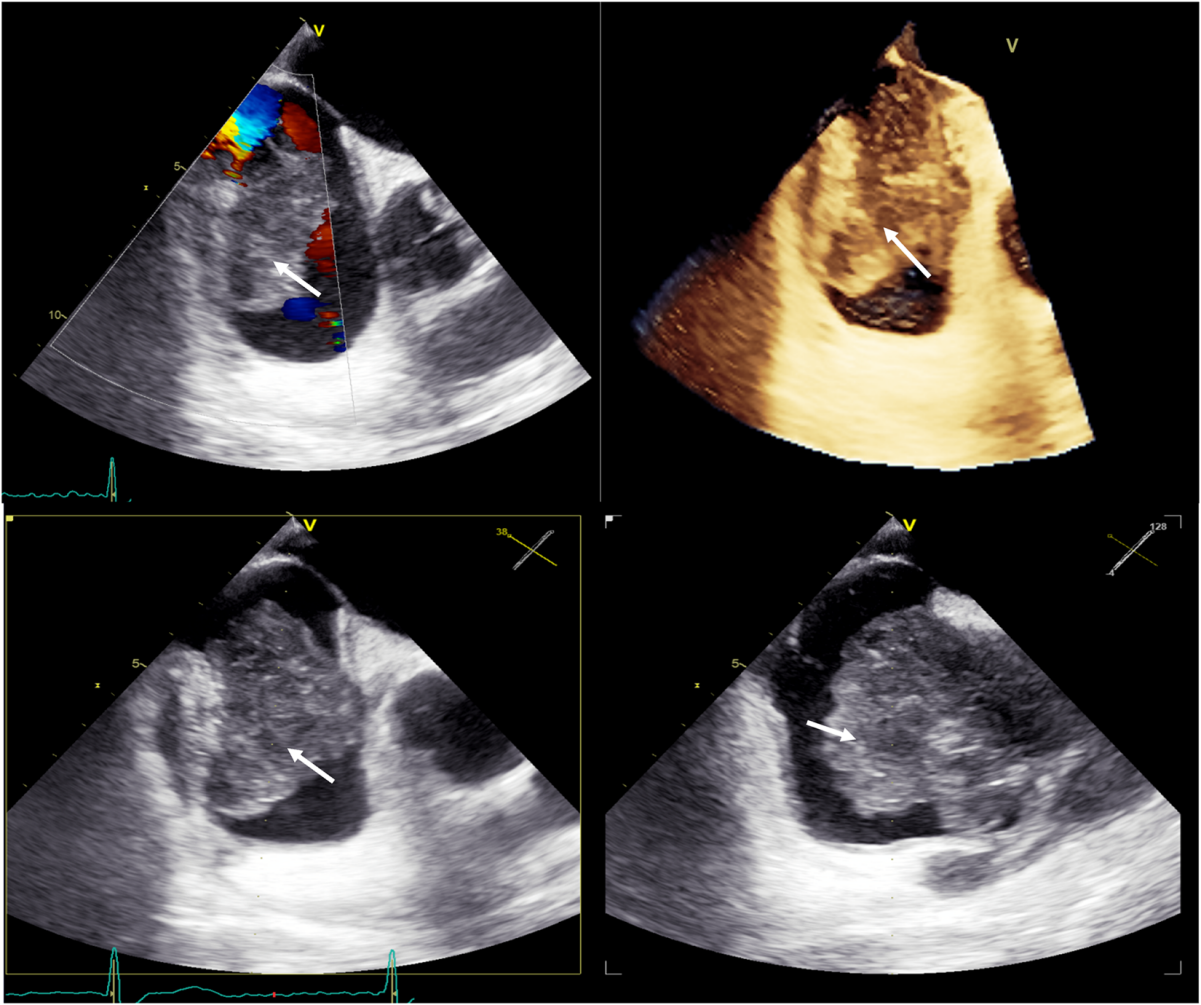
Baseline transesophageal echocardiography with 3D reconstruction, showing a large tumor arising from the lateral wall of the right atrium (white arrows).
